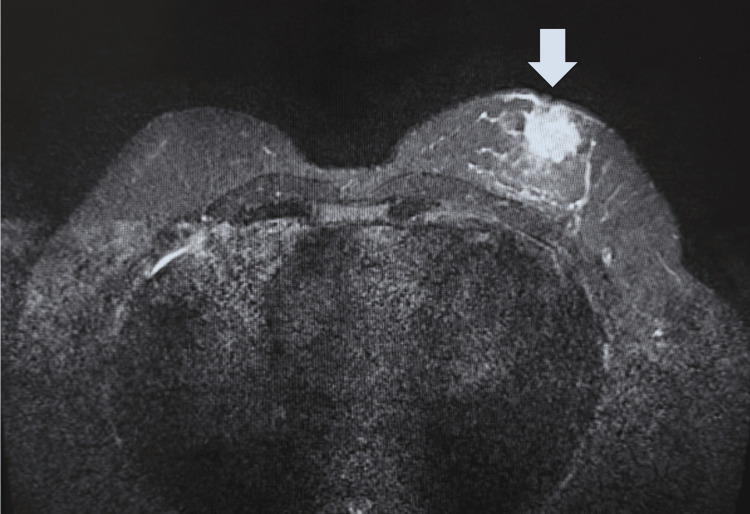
MRI showing a 2.7 x 2.3 x 2.4 cm irregularly shaped enhancing mass (arrow) of the left retroareolar region with rapid uptake, correlating to the biopsy-proven malignancy.
